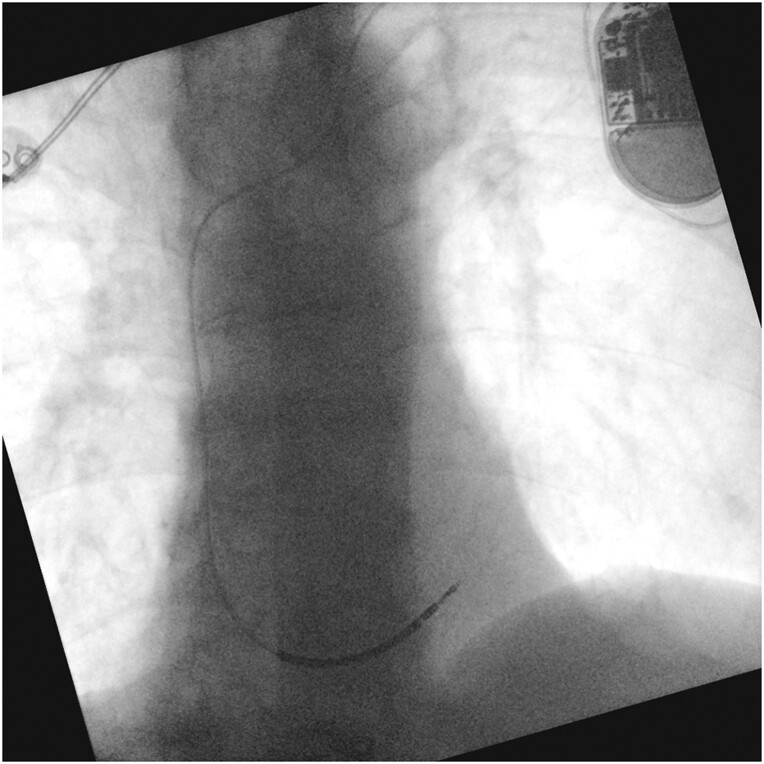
Periprocedural X-ray (anteroposterior view) during the second implantation.
